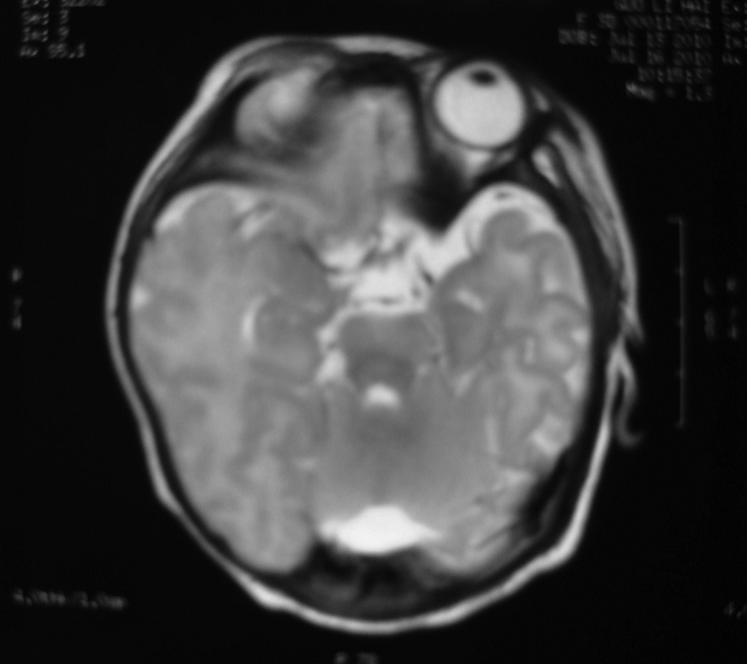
MRI images of neonate head three days after cesarean delivery shows further shrinkage of the torcular thrombosis (T2 weighted images at axial plane).
